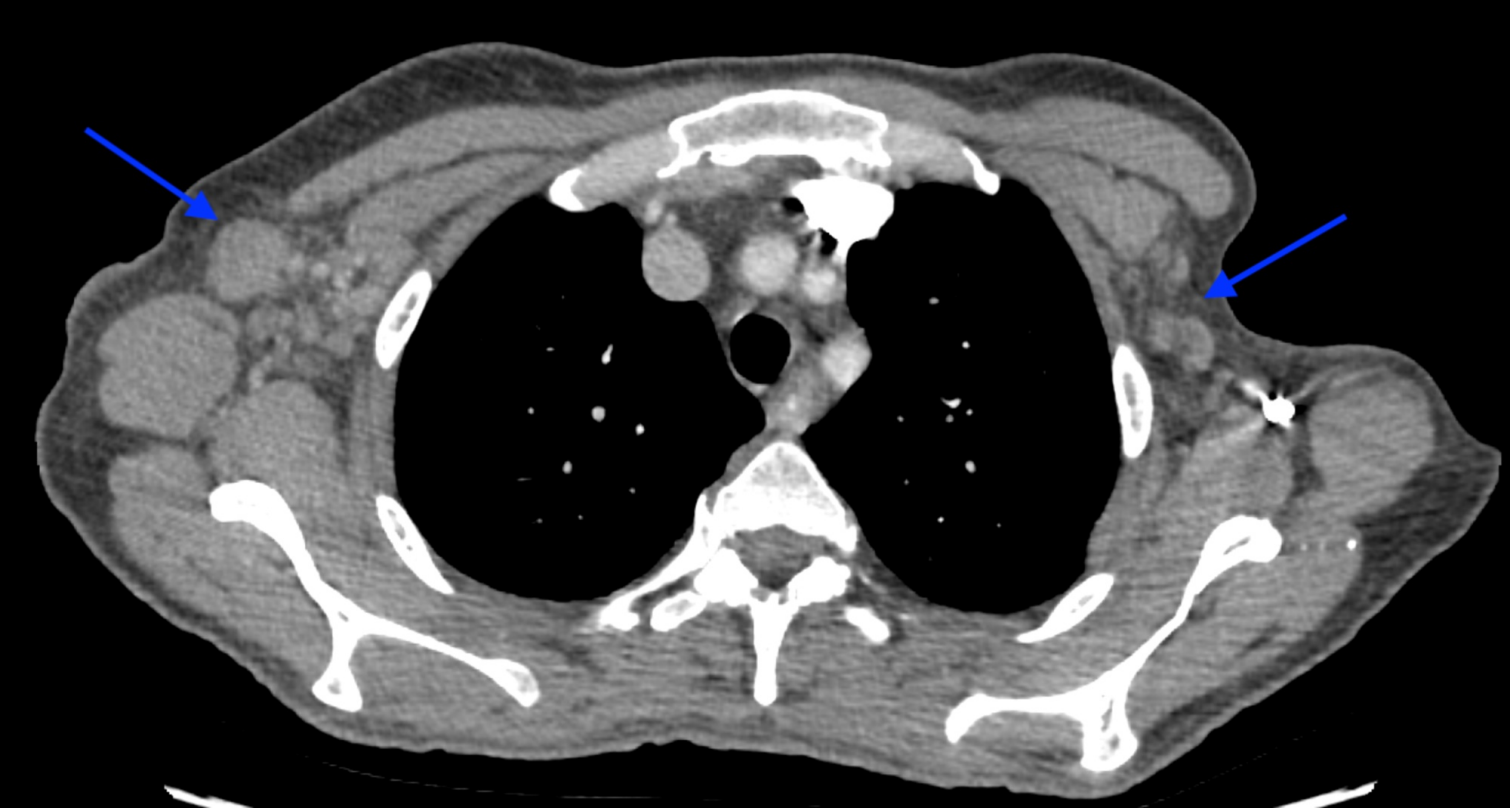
Axial image of the CT scan of chest at the level of axilla shows bilateral axillary lymphadenopathy (blue arrows)
